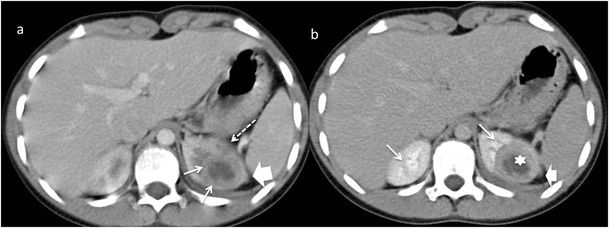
Focal XPN. Same patient as in Fig. 1. a Contrast-enhanced nephrogenic phase transverse CT image shows focal hypodense mass with rim enhancement (*white arrows*) and adjacent parenchymal destruction (*discontinuous white arrow*) in the cortex of the left kidney. Perirenal infiltration (*thick white arrow*) is present. b Contrast-enhanced excretory phase transverse CT image: the lesion is better delimited with thick rim enhancement and hypodense centre (*asterisk*). Working kidneys with contrast material excretion in the collecting system (*white arrows*) are shown. Perirenal infiltration (*thick white arrow*) is present
